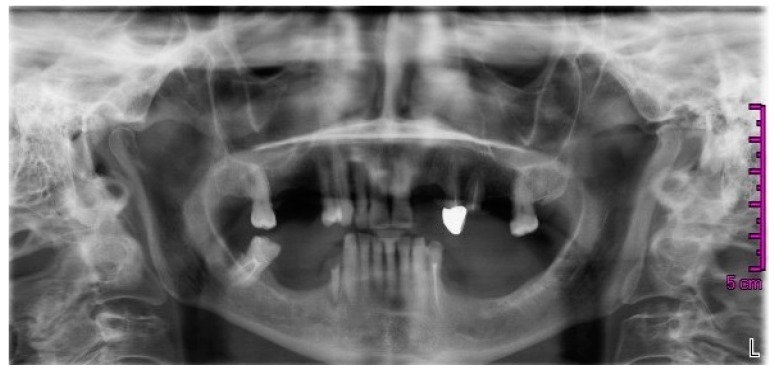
Orthopantomography dated November 2013.
